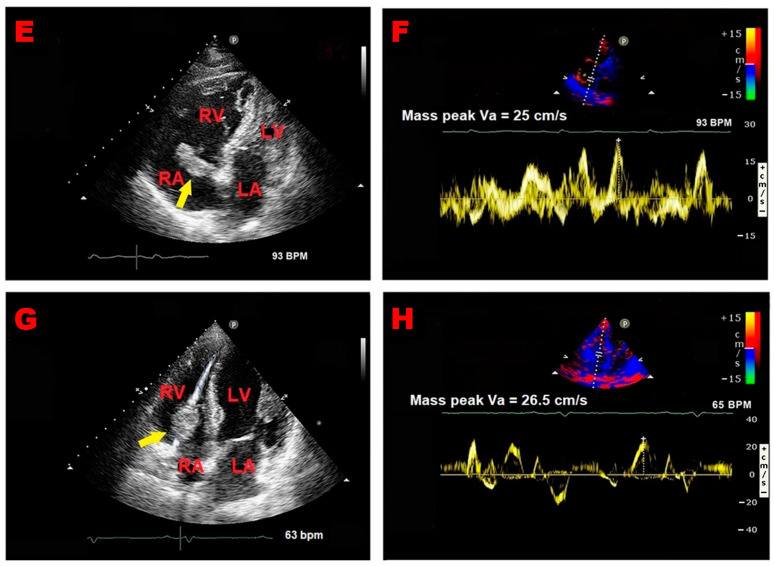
Representative examples of RA thrombus, myxoma, and vegetation assessed by TTE implemented with PW-TDI. (A) Transthoracic echocardiography. Apical four-chamber view, showing large S-shaped RA thrombus (yellow arrow) entrapped in the Chiari network, prolapsing through the tricuspid valve into the right ventricle. (B) PW-TDI assessment of the thrombotic mass motility: the pattern of incoherent motion is typical of a pathological RA mass. (C) Transthoracic echocardiography. Apical four-chamber view, revealing RA atrial multilobulated, hypermobile, echogenic cauliflower mass attached to the tricuspid lateral annulus with a short stalk (yellow arrow), compatible with a pedunculated myxoma. (D) PW-TDI assessment of the RA myxoma motility: the mass motility is totally independent of the cardiac cycle. (E) Transthoracic echocardiography. Apical four-chamber view, demonstrating an echogenic mass attached to the fossa ovalis, extending into the RA (yellow arrow), compatible with RA myxoma. (F) Pattern of uncoordinated motion of RA myxoma assessed by PW-TDI. (G) Transthoracic echocardiography. Apical four-chamber view, highlighting large vegetation attached to the pacemaker lead in the right atrium (yellow arrow) of a patient with infective endocarditis. (H) Pattern of incoherent motion of RA vegetation on PW-TDI. LA, left atrium; LV, left ventricle; PW, pulsed wave; RA, right atrium; RV, right ventricle; TTE, transthoracic echocardiography; TDI, tissue Doppler imaging; Va, antegrade velocity. (C,D) are reproduced from the paper [24] (license number 5917070978039).
